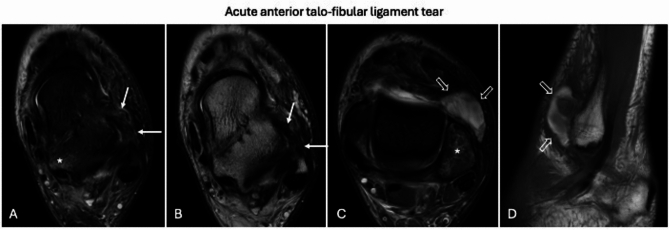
Acute anterior talo-fibular ligament partial tear. Ankle MRI performed 18 days after trauma shows edematous and thickened appearance of anterior talo-fibular ligament (A, B; white arrows) with peri-capsular edema, talus (A) and lateral malleolus (C) bone edema (asterisks). Also note the anterolateral hematoma (void arrows) with high signal on axial fat-suppressed proton density weighted (C) and sagittal T1-weighted (D) images
